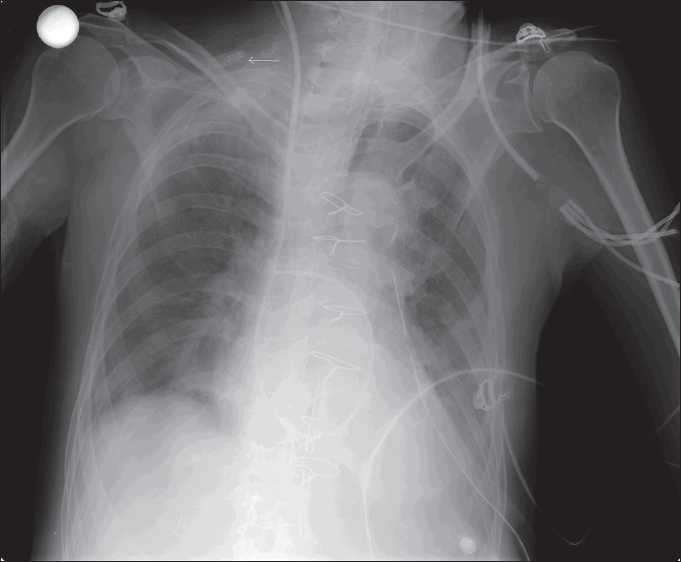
Chest radiograph showing metallic object above the right clavicle
